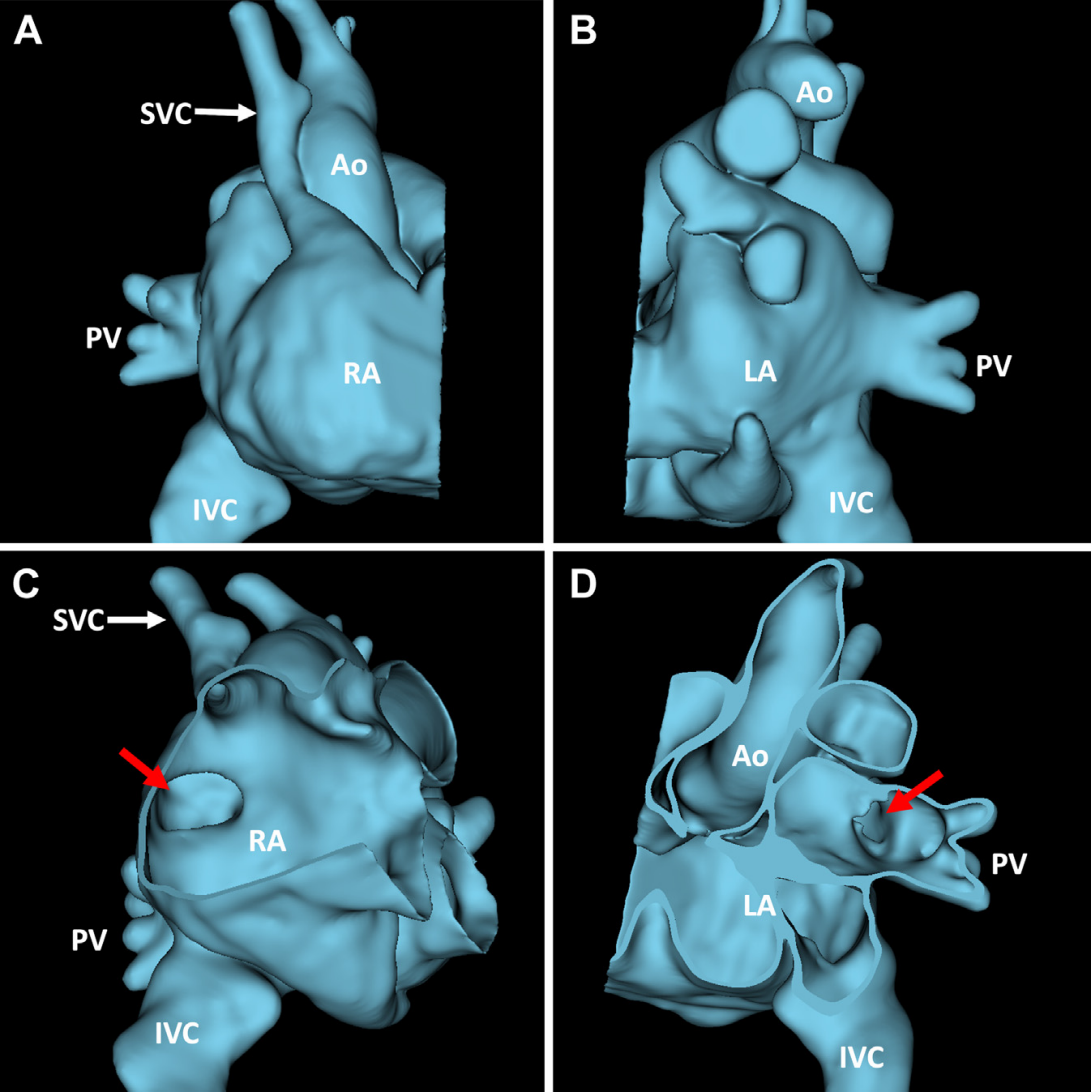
CMR-Based Modeling to Inform 3D Echocardiographic Views (A) Right lateral view of heart segmentation. (B) Left lateral view of heart segmentation. (C) Right lateral view of segmentation displaying site of patch dehiscence (red arrow). (D) Left lateral view demonstrating site of patch dehiscence (red arrow). RA = right atrium; LA = left atrium; PV = pulmonary veins; IVC = inferior vena cava Ao = aorta.
